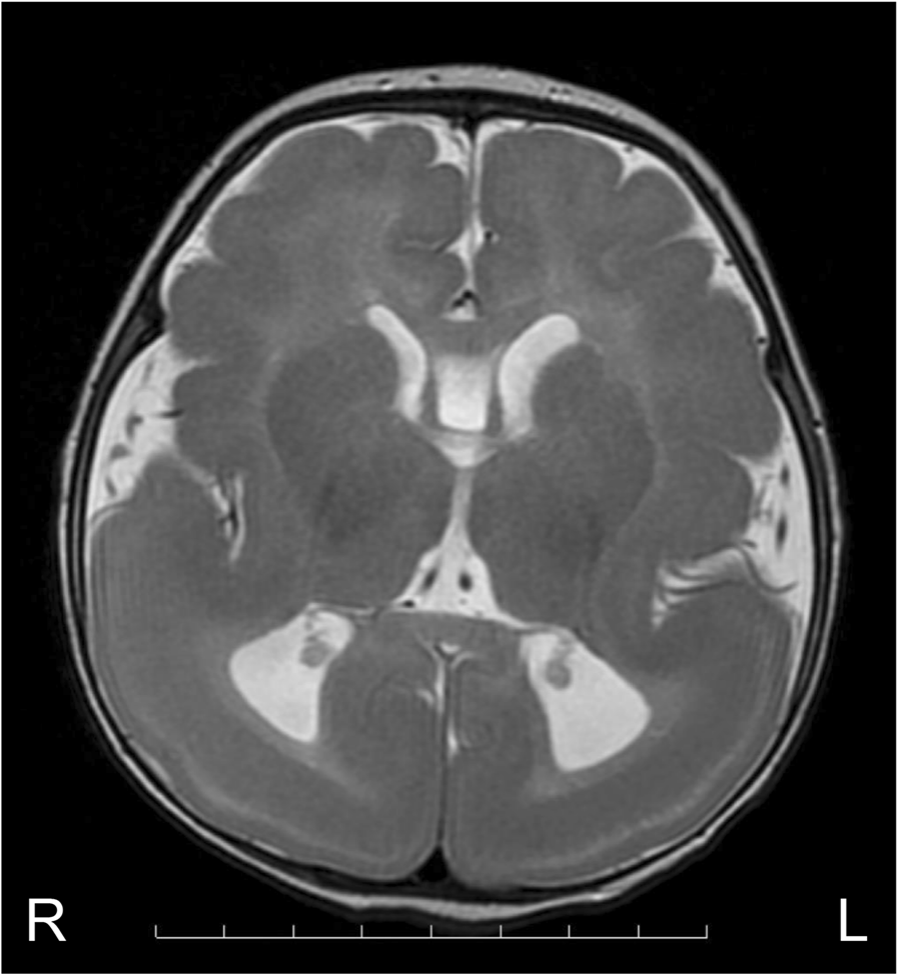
Brain MRI scan of a patient with an intragenic tandem duplication in the *PAFAH1B1* gene. Axial T2-weighted brain image indicating the occurrence of predominant agyria in the posterior regions, and pachygyria in the anterior regions. The patient was diagnosed with grade 3 lissencephaly
